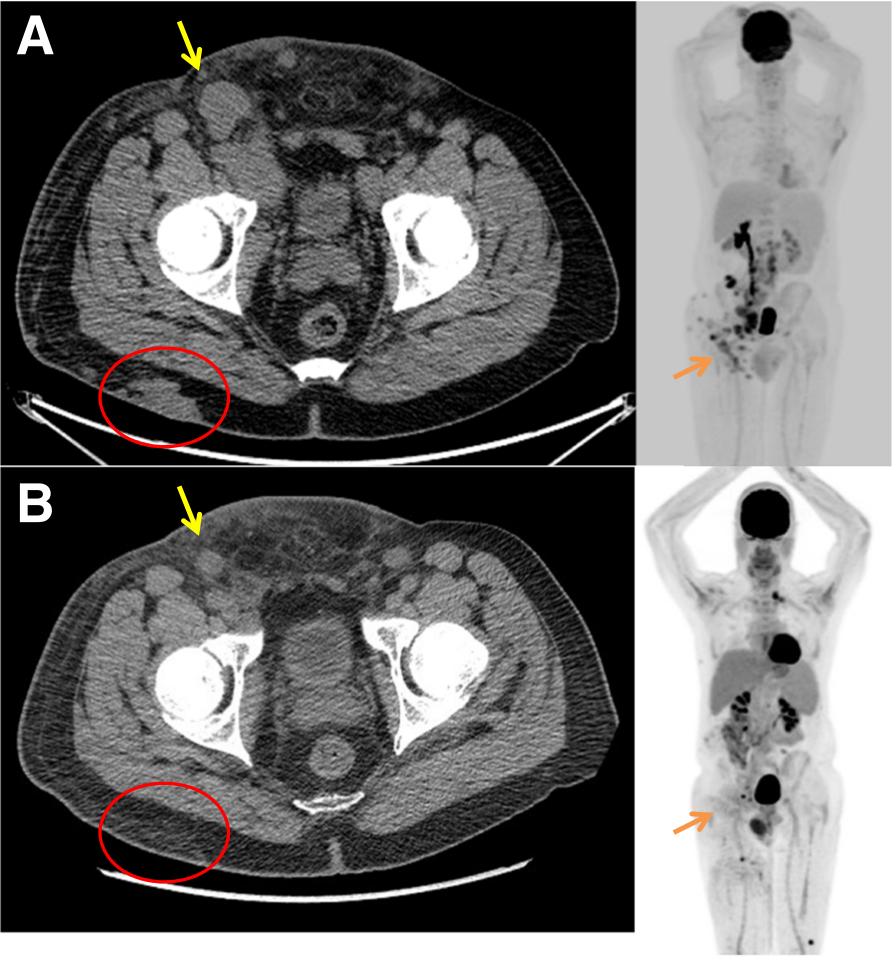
Computed tomography at the level of the pelvis and maximum intensity profile of 18-fluorodeoxyglucose positron emission tomography demonstrates the primary Merkel cell carcinoma tumor of the right buttock (red circle), as well as in-transit metastases and lymphatic metastases (yellow arrow) before (a) and after (b) radiotherapy. A partial response was noted in the irradiated tumors, with progression of metastases elsewhere
